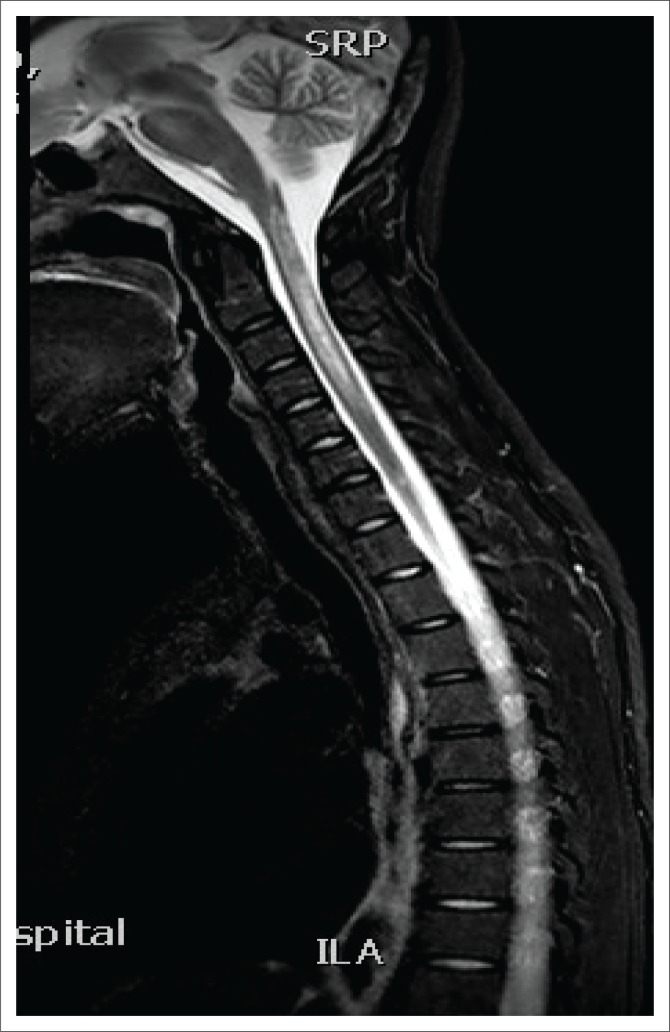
Sagittal short TI inversion recovery magnetic resonance images demonstrating longitudinally extensive spinal cord lesion.
